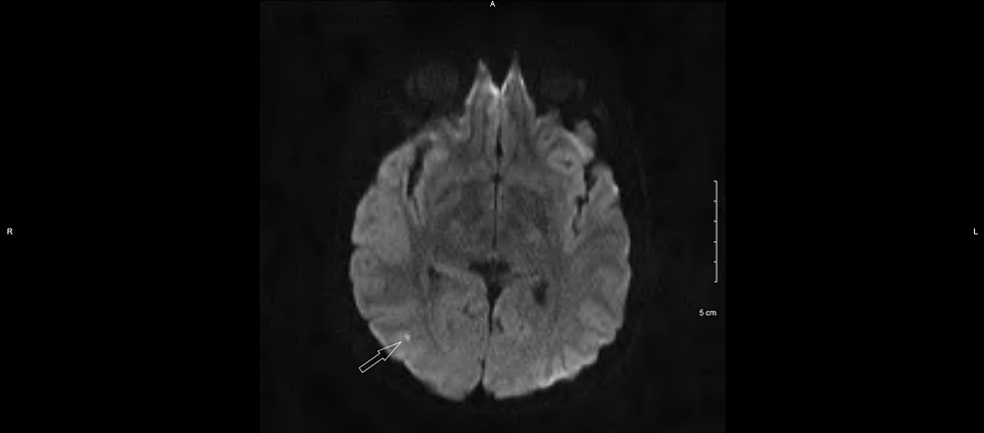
MRI of the brain showing a hyperintense lesion in the right parietal-occipital region
